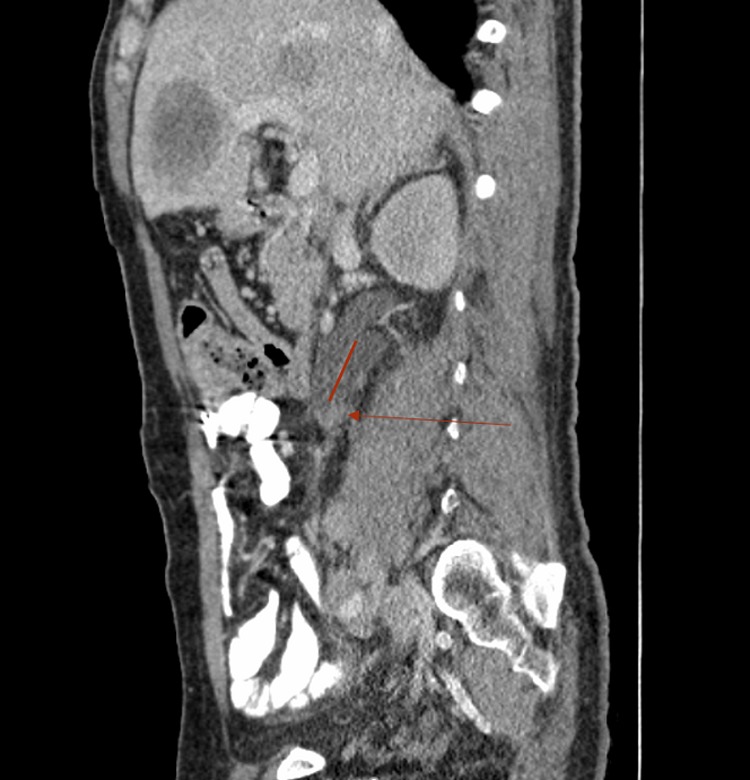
Sagittal view of CT scan with contrast showing a hydronephrotic bifid ureter (*red line* indicating boundary between two ureteral segments) with enhancement at bifurcation (see *arrow*).
